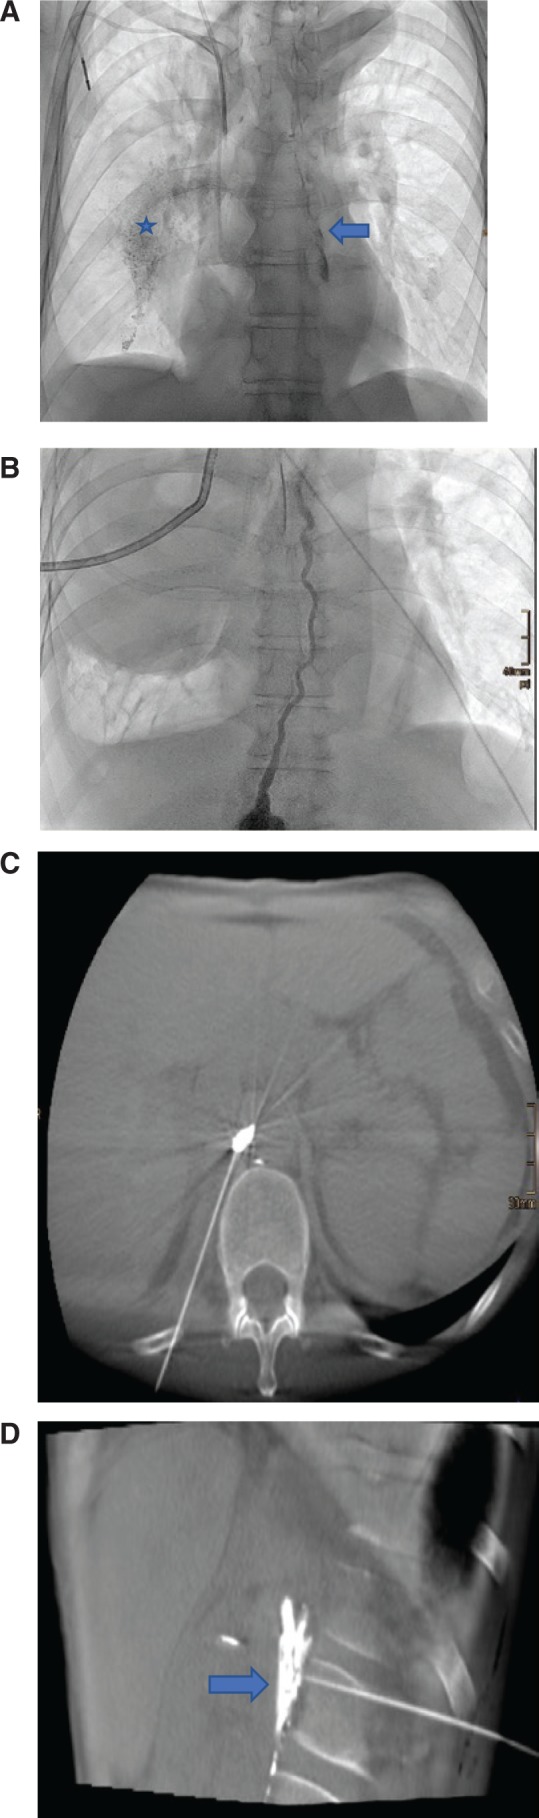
Radiologic intervention with detection and treatment of chylous leak. (A) Spot radiograph of the thorax showing leakage site at the mediastinum (arrow). Radiopaque Lipiodol accumulates in the pleural space on the right side (asterisk). (B) Spot radiograph showing the course of the thoracic duct in another patient. A right-sided chest drain was placed to evacuate a chylous pleural effusion. (C) Cone beam CT-guided puncture of the cisterna chyli. (D) Parasagittal multiplanar reformation shows the “needle disruption technique.” If the thoracic duct cannot be successfully cannulated for embolization after needle placement, multiple needle passes can be used to macerate the cisterna chyli. This promotes chyle flow into retroperitoneum, as shown by the extravasation (arrow), thereby allowing healing of the leak in the mediastinum.
